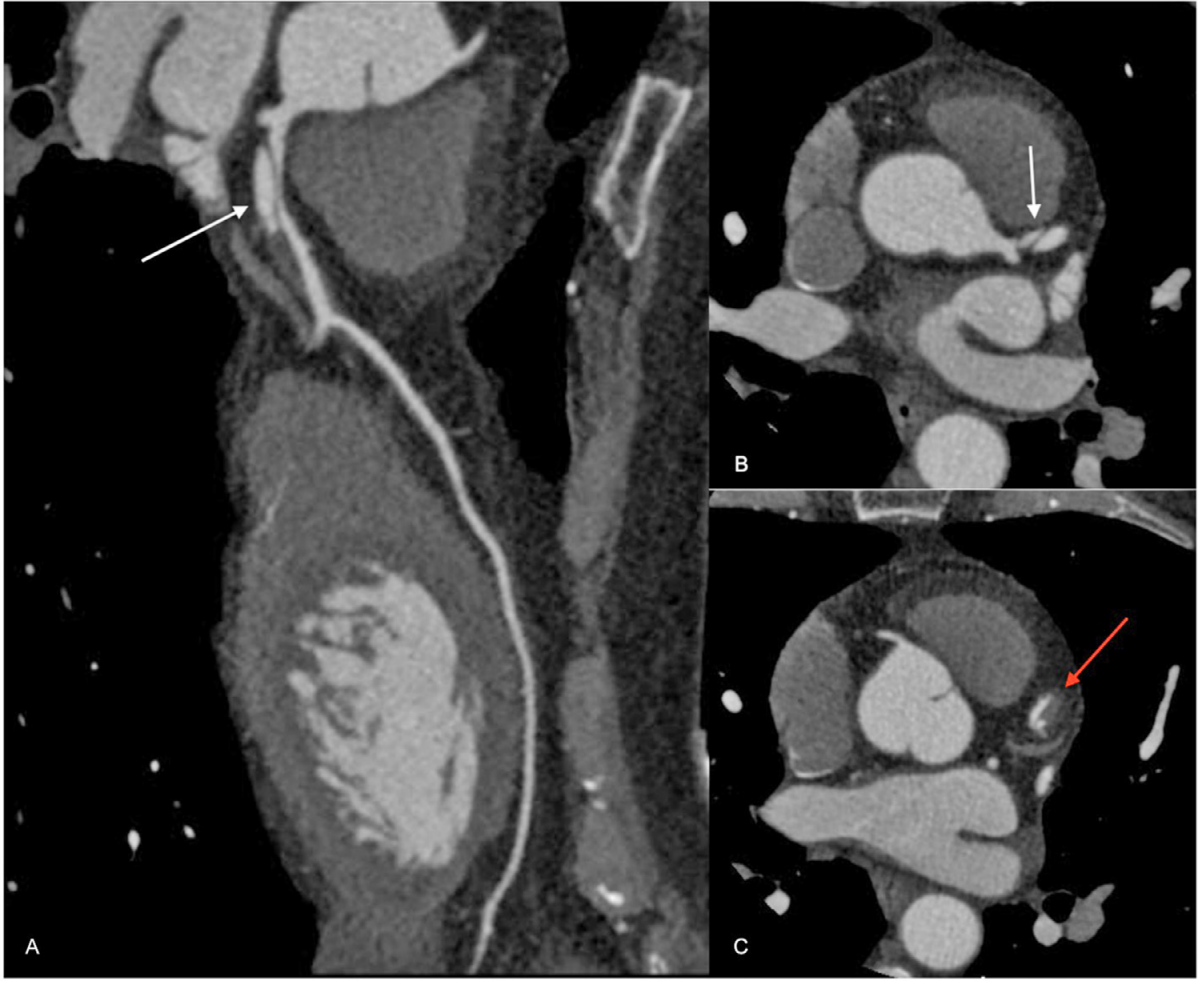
Coronary CT scan images showing dissection of LAD. The white arrows in the oblique (A) and in the axial (B) images focus on the dissection flap of the proximal and middle tract of the vessel causing narrow of the true lumen, whereas the red arrow in the second axial image (C) shows the partial thrombosis of the false lumen.
